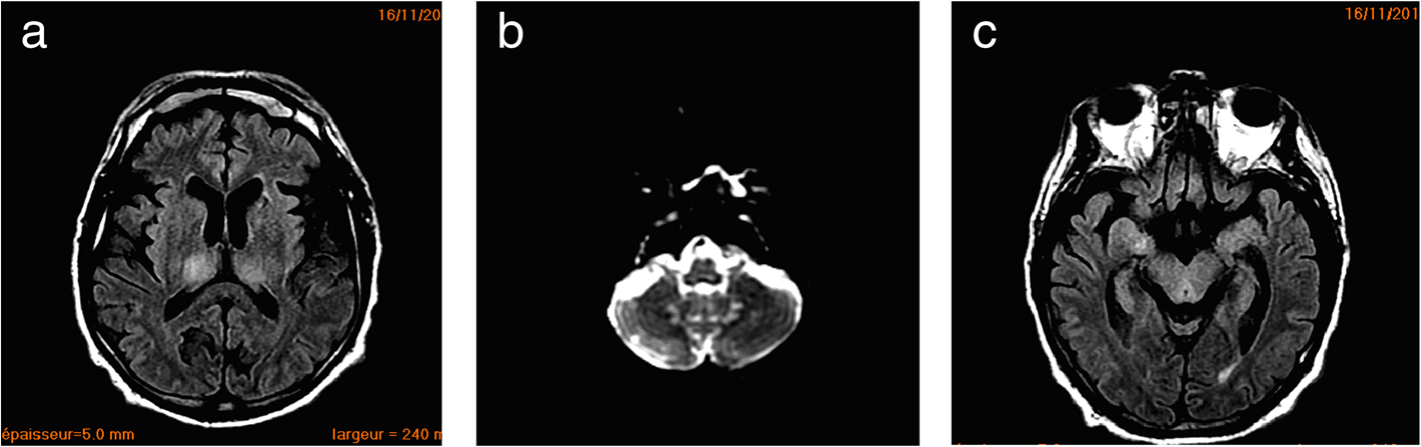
Brain abnormalities of a patient. (a) MRI showing hyperintense lesions of thalami. (b) Same patient; MRI showing hyperintense lesions of dentate nuclei. (c) Same patient; MRI showing lesions of the posterior white matter in the brainstem pons and in the periaqueductal gray matter of the midbrain.
